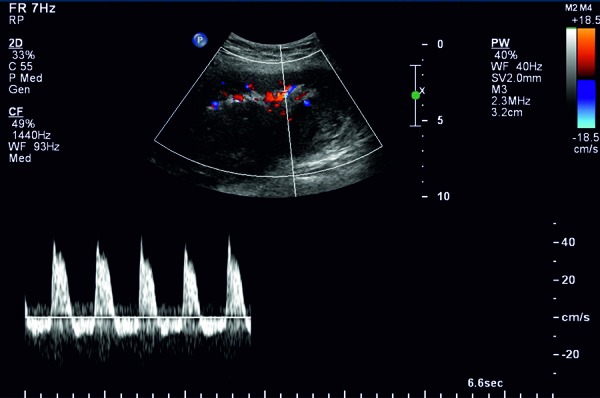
Duplex ultrasound showing reversal of diastolic blood flow in intrarenal artery.
